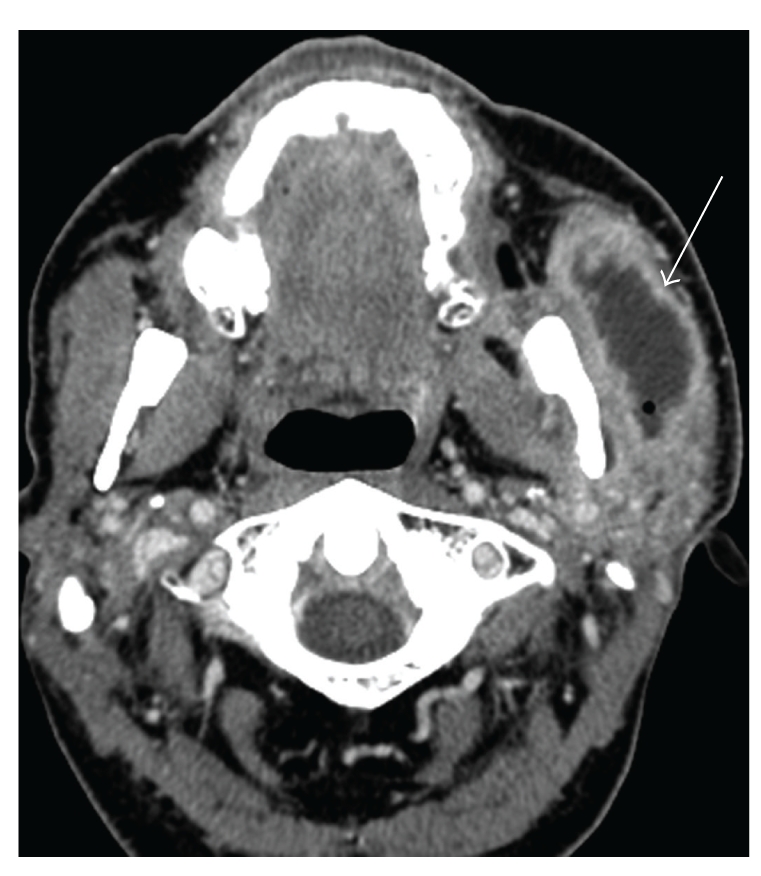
Left facial abscess: (a) Axial contrast-enhanced CT (soft tissue window) shows an abscess (arrow) of the left face. This is characterized by a peripherally enhancing fluid collection with a small focus of air and adjacent soft tissue swelling.
